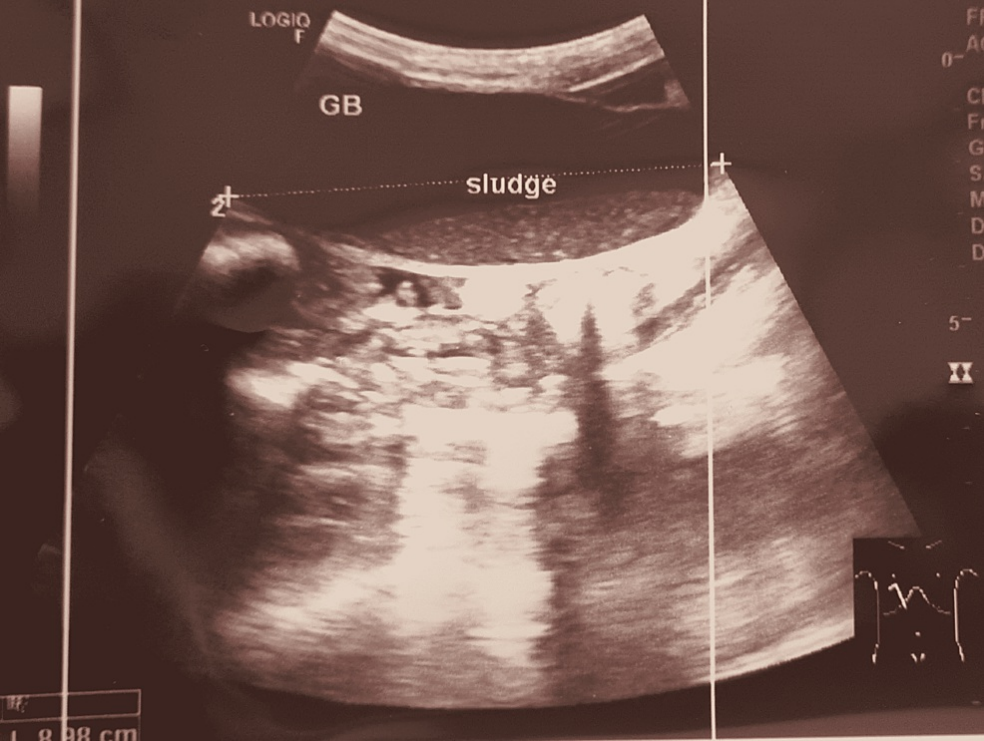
Gallbladder hydrops and bile sludge on abdominal USG. USG: ultrasonography
